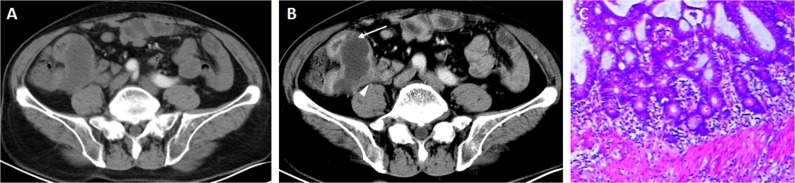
Contrast enhanced CT scan showed cystic mass with mild ring mural enhancement in the arterial phase (A). Progressive enhancement of mini mural nodule (arrow), and a few amounts of periappendiceal fat stranding (arrow head) were noted in the portal venous phase (B). The mucinous epithelium was remarkably bland with small nuclei with no signs of invasive growth (C).
